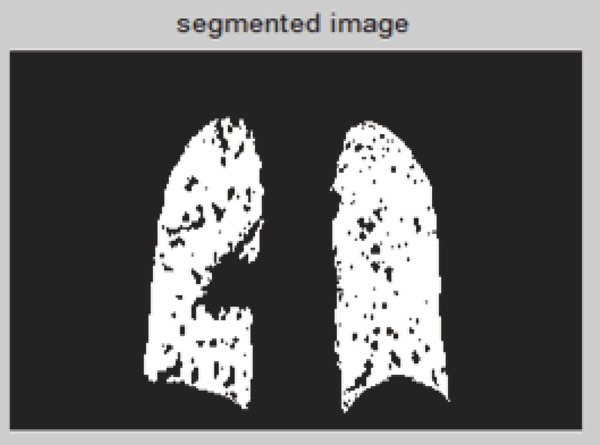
Thresholding approach of 4D CT image.
